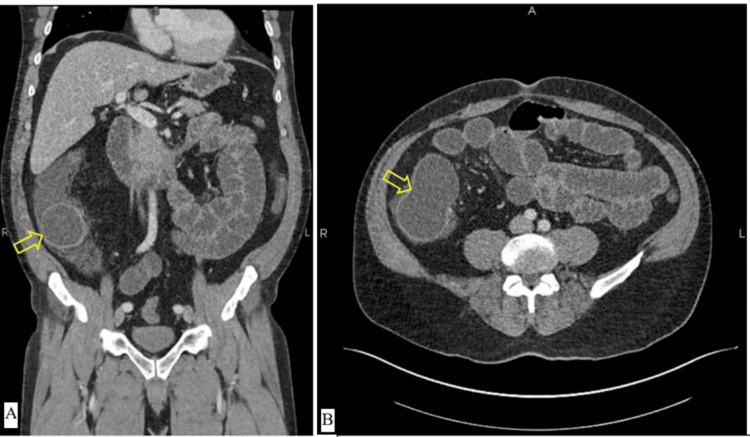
Preoperative computed tomography imaging with intravenous contrast of the abdomen and pelvis (A: coronal; B: axial) showing a 9.3 x 4.9 x 5.4 cm lobulated cystic mass at the ileocecal transition point, indicated by yellow arrows
